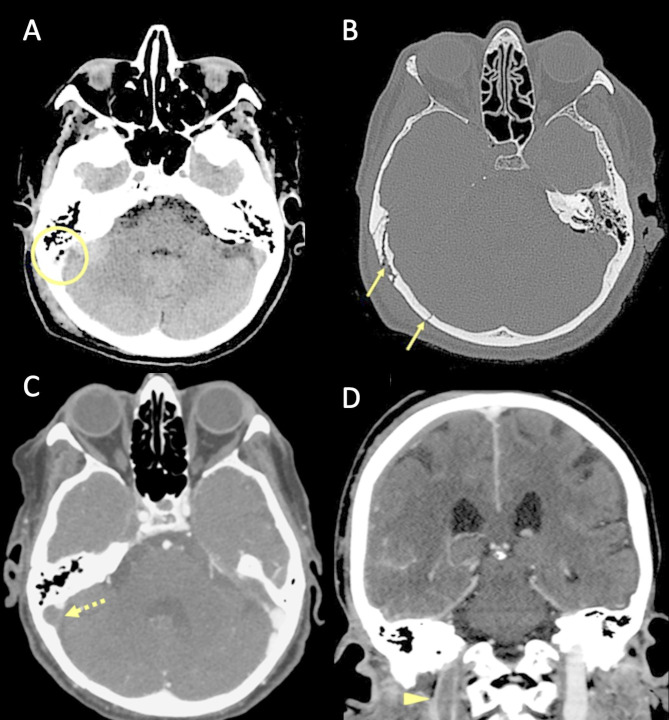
Blunt trauma in a patient at the beginning of the ninth decade of life. Non-enhanced CT scan (A, B) obtained at presentation shows the presence of air bubbles and hyperdensity of the right transverse sinus (A, circle), adjacent to a diastasis of the occipito-mastoid suture, with a fracture of the ipsilateral squamous occipital bone (B, arrows). Follow-up CTA performed after 48 h (C, D), shows a lack of opacification of the right transverse sinus (C, dotted arrow) and of the ipsilateral jugular vein (D, arrowhead), consistent with venous thrombosis
